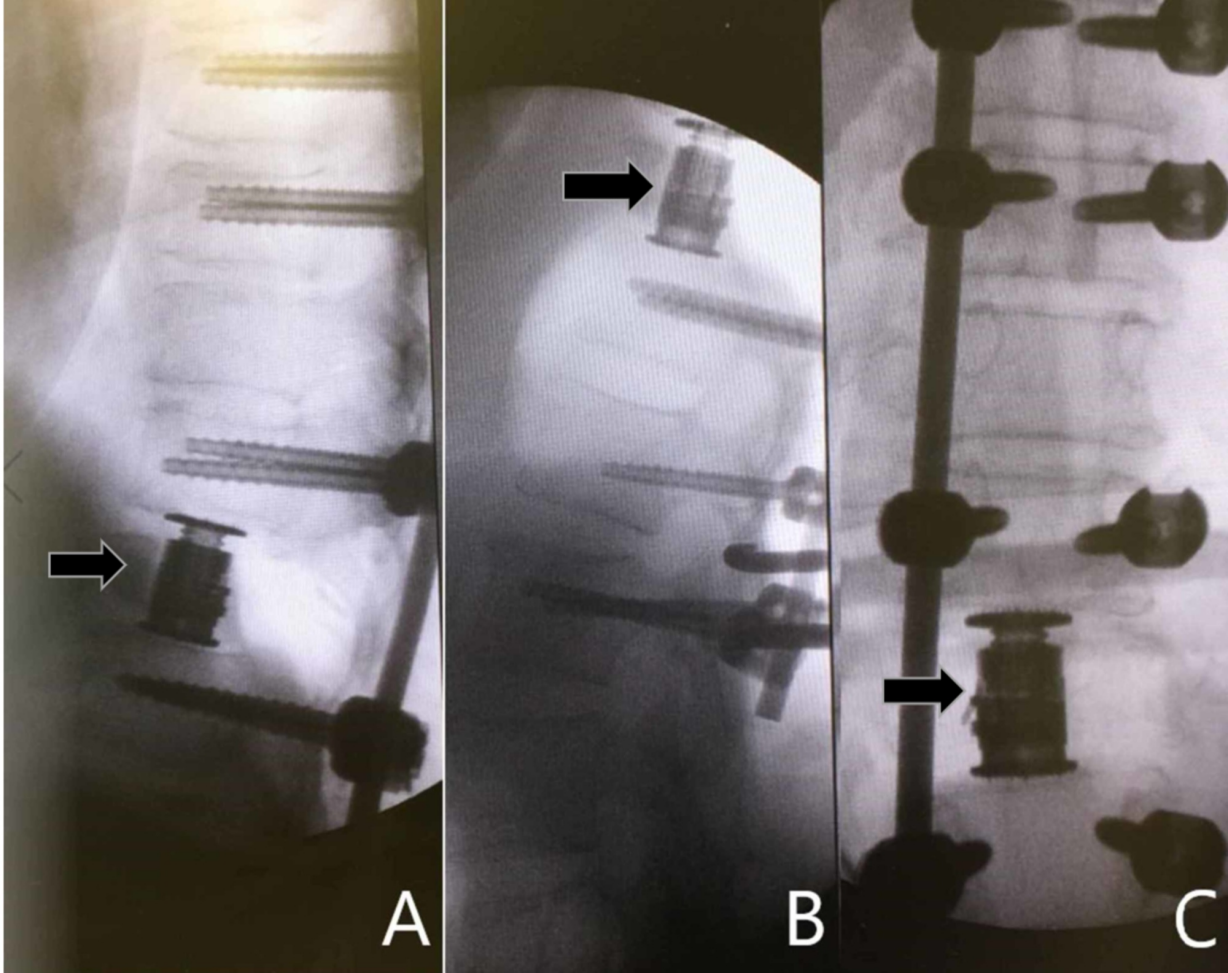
Intra-operative fluoroscopic lateral (A and B) and AP (C) X-ray images demonstrate pedicle screw placement bilaterally at T7, T8, T10, T12, L1, and L2 with an expandable interbody cage placement at T11 (black arrow). AP, anteroposterior
